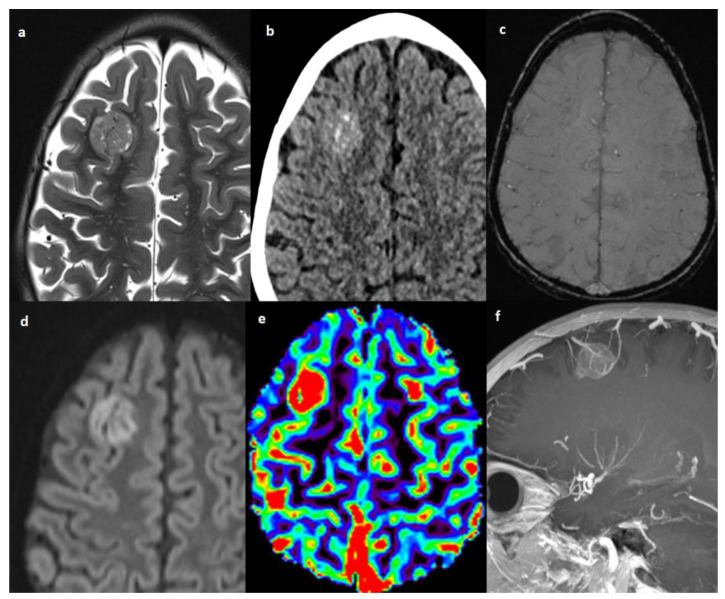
Patient #4 imaging features. Axial T2w image (a) shows a single lesion in the right frontal lobe, hyperdense on axial non contrast brain computed tomography (CT) (b), without hemorrhagic components in susceptibility weighted imaging (SWI) (c), but with restricted diffusion in diffusion weighted imaging (DWI) (d). The cerebral blood volume map from dynamic susceptibility contrast (DSC) perfusion images (e) shows elevated relative cerebral blood volume (rCBV) within the lesion consistent with angiogenesis/vascular proliferation. Post-contrast sagittal T1w image with maximum intensity projection (MIP) reconstruction (f) shows tortuous vessels within and around the lesion.
